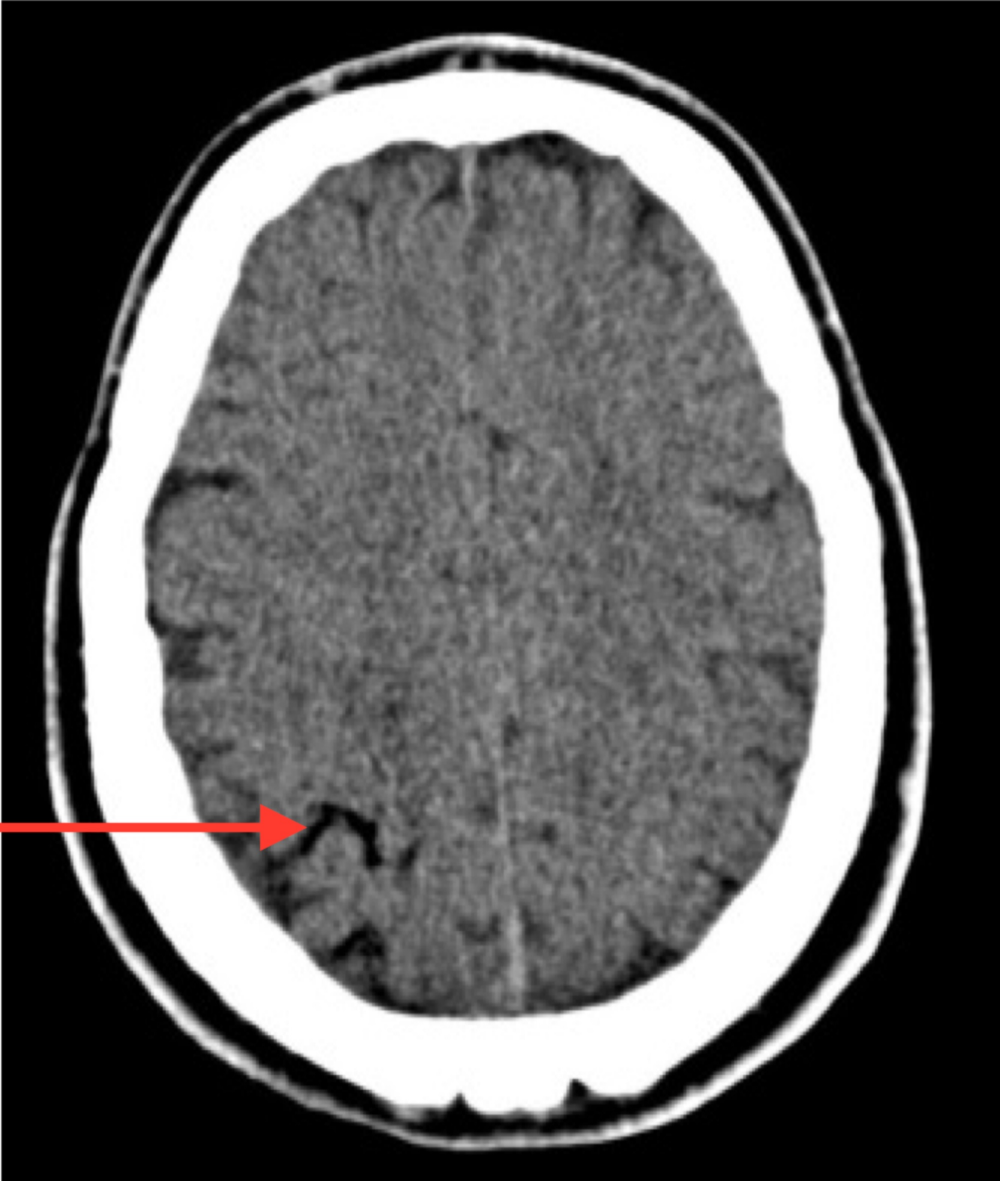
Coronal slice of computed tomography (CT) of the brain with a red arrow demonstrating intraparenchymal air
